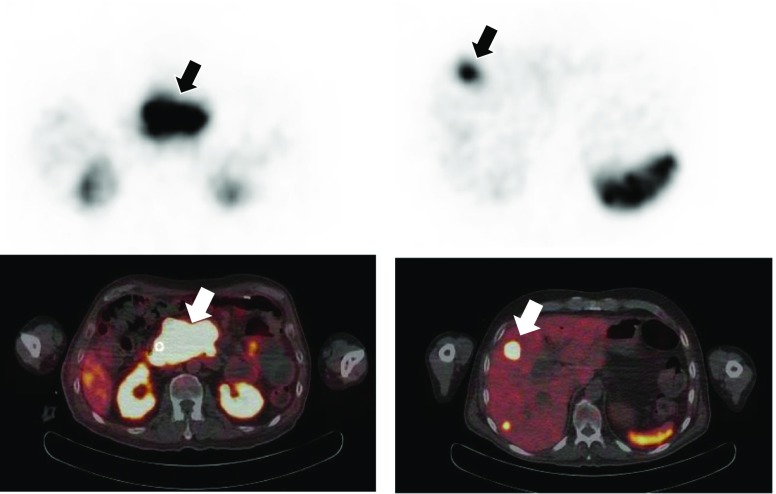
^111^In-DOTATATE SPECT and ^68^Ga-DOTATATE PET/CT images of neuroendocrine tumours in a patient treated with ^90^Y-DOTATATE radiopeptide therapy. *Arrows* indicate the lesions for which doses are to be calculated
